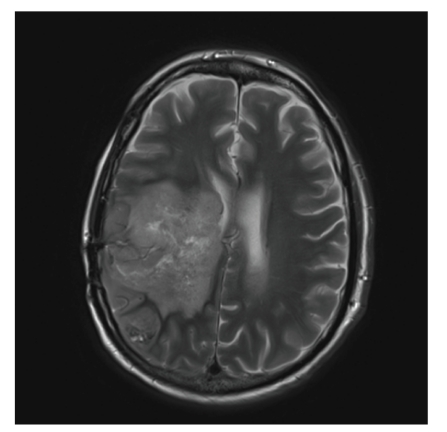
MRI of the brain, 6 weeks after embolectomy, showing stable disease.
